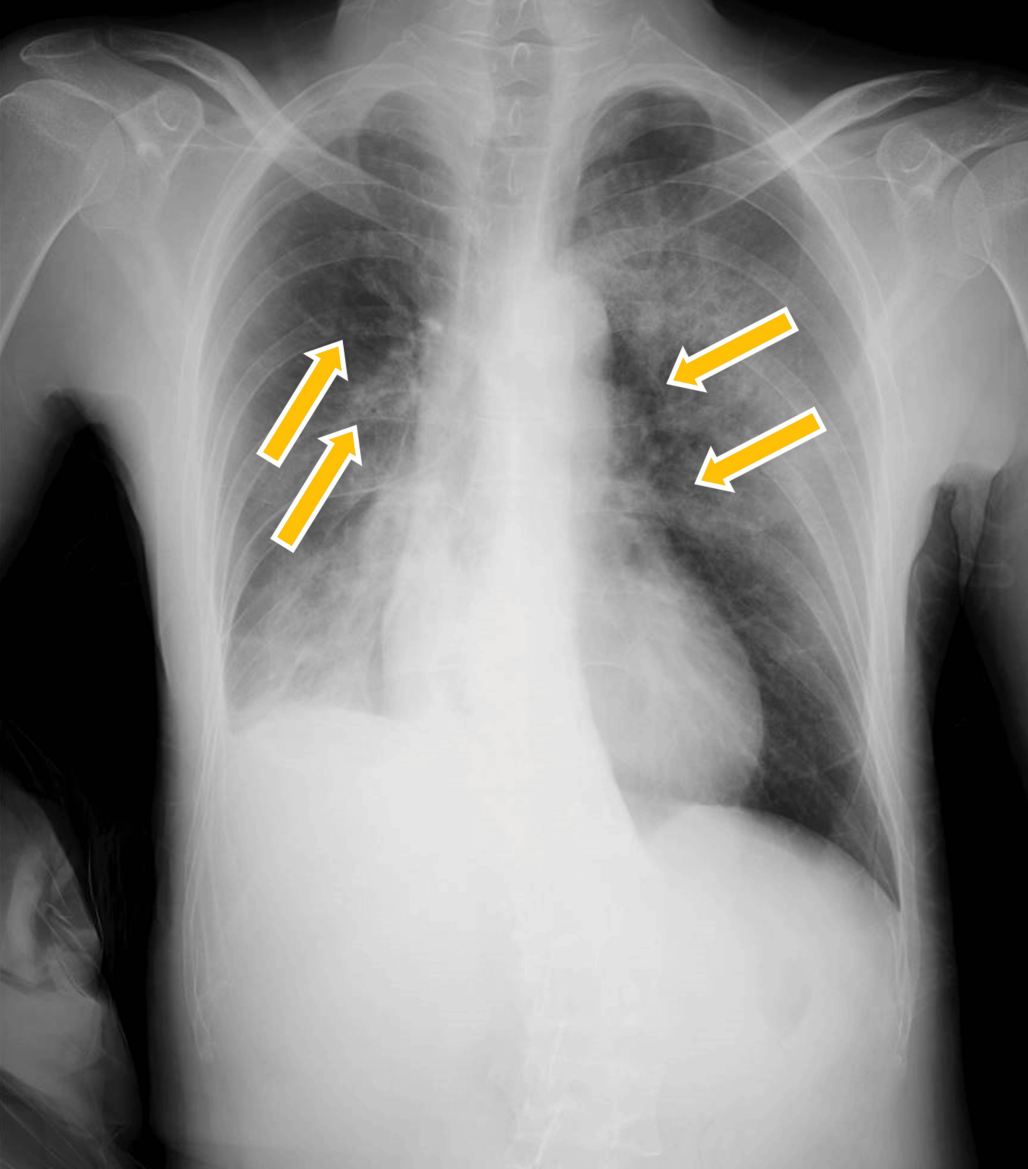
Preoperative chest radiograph. The orange arrows indicate multiple fractures of the ribs
